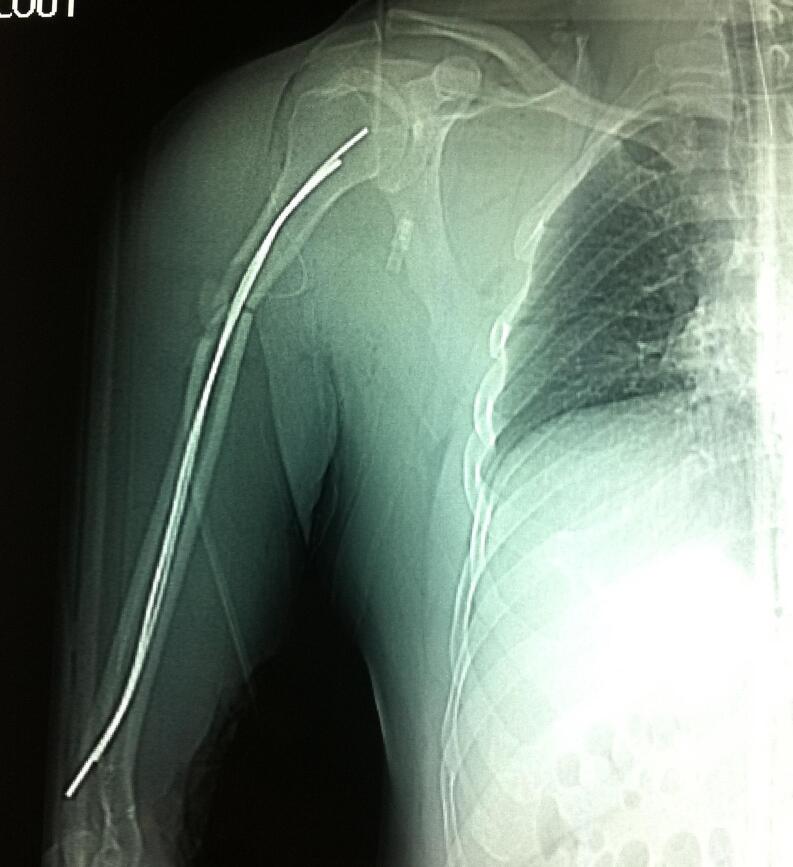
Post-operative X-rays after reduction and Hackethal bundle nailing.
